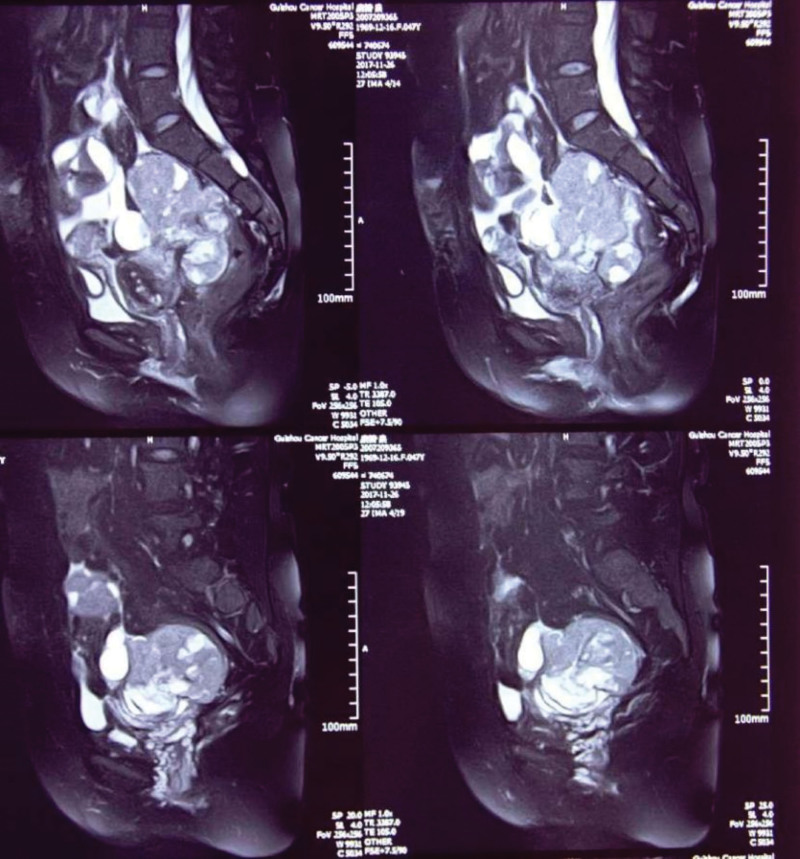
Pelvic magnetic resonance imaging showed a huge cystic-solid space from the pelvis to the lower abdomen, with intratumoral necrosis, hemorrhage, and cystic degeneration, and multiple space-occupying shadows on both sides of the incision after surgery on the anterior abdominal wall of the lower abdomen (December 2017).
